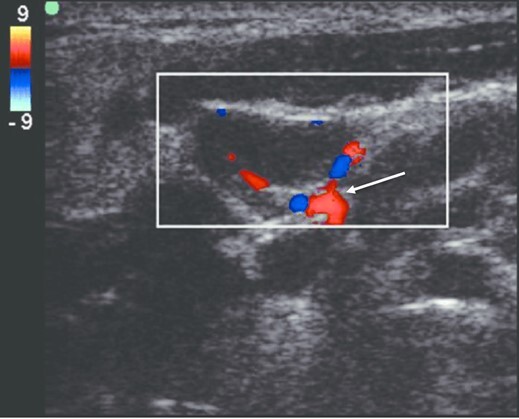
White arrow points to point-of-care-ultrasound color Doppler image of benign cervical lymph node showing hilar/central vascularity.^15^
